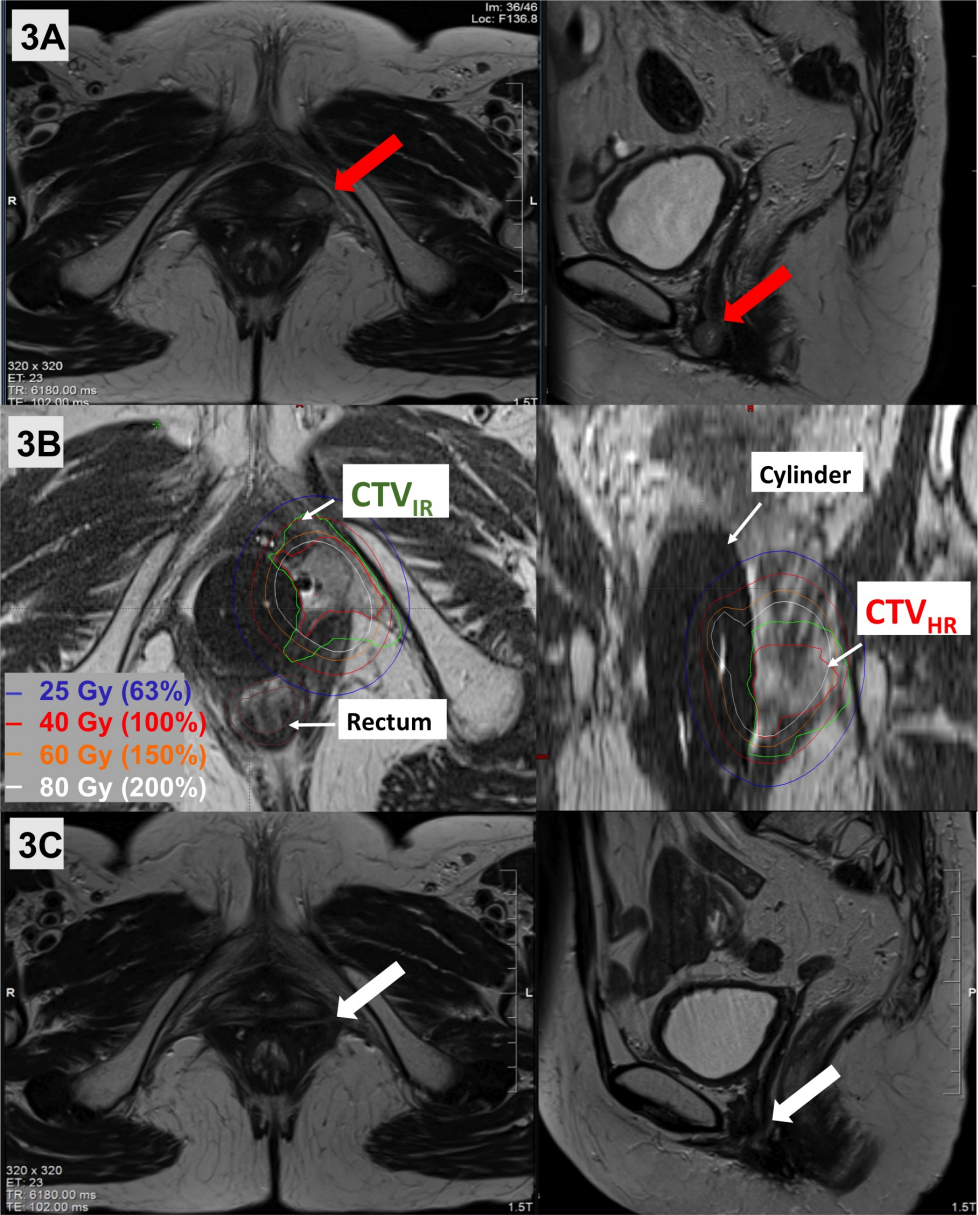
(A) Representative T2-weighted axial and sagittal images of Patient B’s lower vaginal recurrence (red arrows). (B) MR-guided salvage brachytherapy treatment was delivered. using a customized vaginal mold and oblique needles, with excellent coverage of the GTVres (magenta line), CTVHR (overlaps with GTVres) and CTVIR (green line). (C) MRI at 35 months post-brachytherapy showed no residual disease (white arrows). CTV_HR_ = High risk clinical target volume; CTV_IR_ = Intermediate risk clinical target volume; GTV_res _= Residual gross tumor volume at brachytherapy
